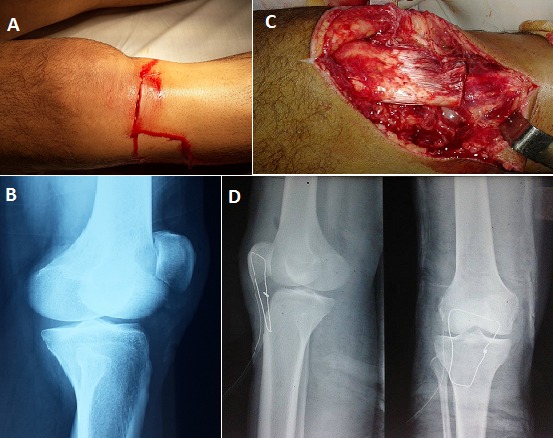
(A): image montrant la plaie pénétrante du genou; (B): radiographie standard du genou en incidence de profil montrant l'ascension de la patella; (C): vue per-opératoire montrant la section complète du tendon patellaire; (D): radiographie standard du genou en incidence de face et de profil montrant le cadrage de protection de la suture tendineuse
